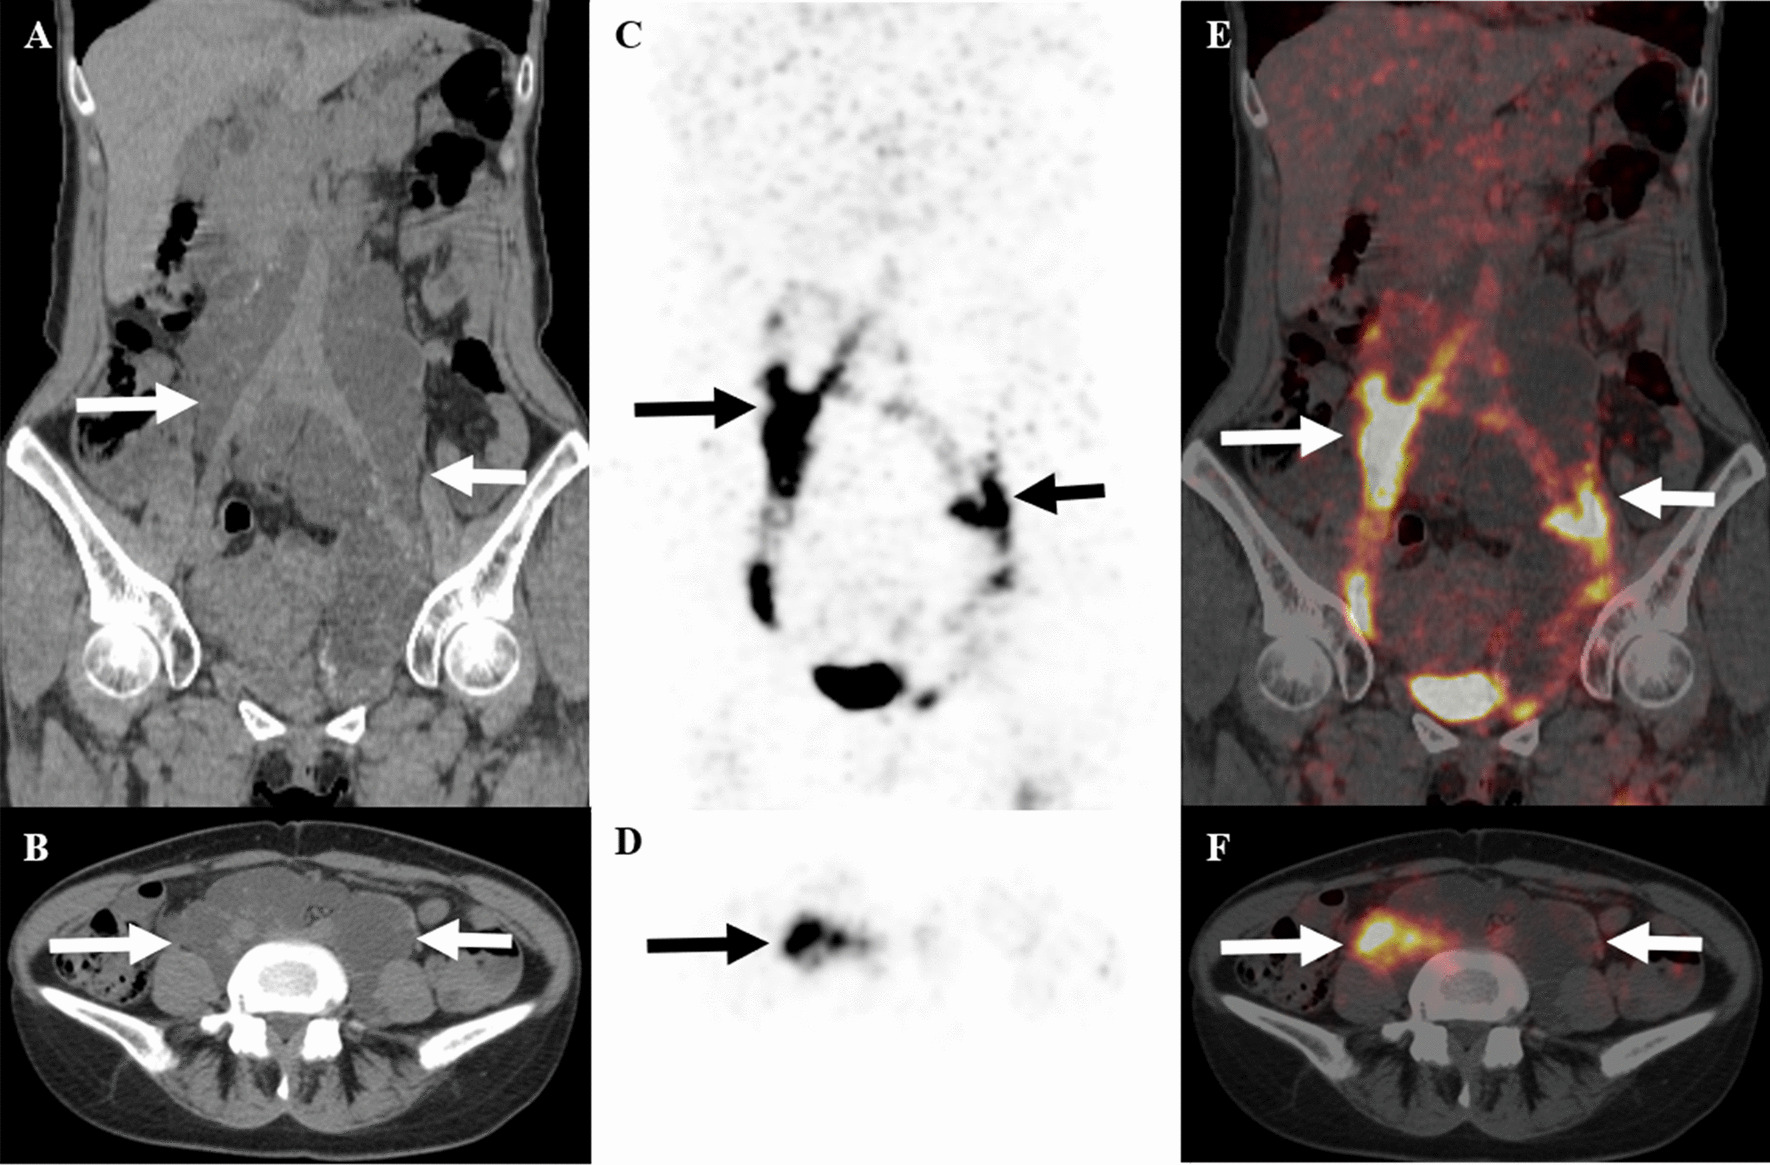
A 48-year-old woman (patient #8) with LAM. Co-registered CT scans (A, coronal view, B: axial view; white arrows) of ^68^Ga-NEB PET/CT demonstrated a large hypodense multiloculated cystic mass extending from the retroperitoneum into the pelvis, consistent with lymphangioleiomyoma. On ^68^Ga-NEB PET (C, coronal view; D, axial view; black arrows) and fusion (E, coronal view; F, axial view; white arrows) images, the tracer was only seen in part of the cystic components
